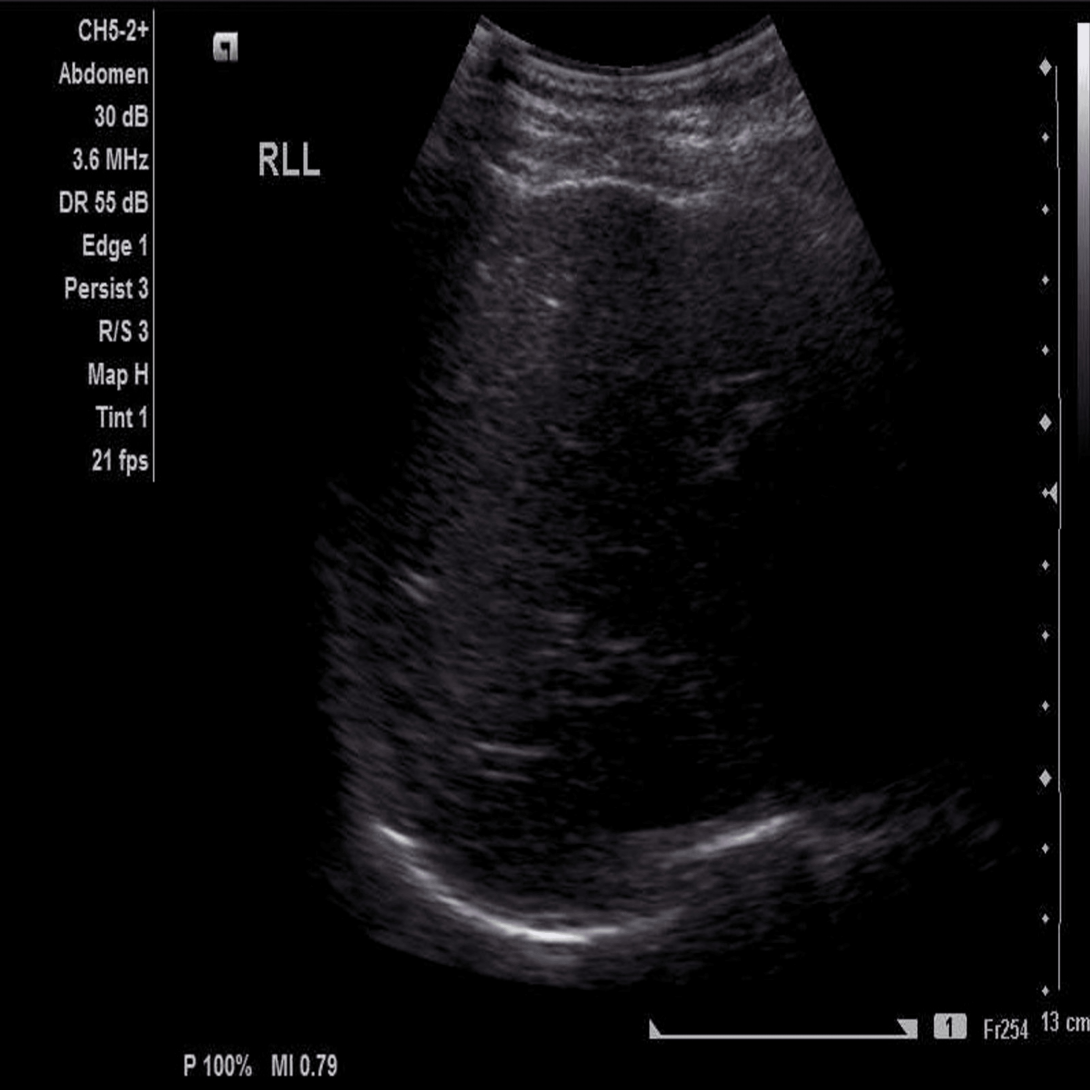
Ultrasound of the abdomen revealing normal liver size.
